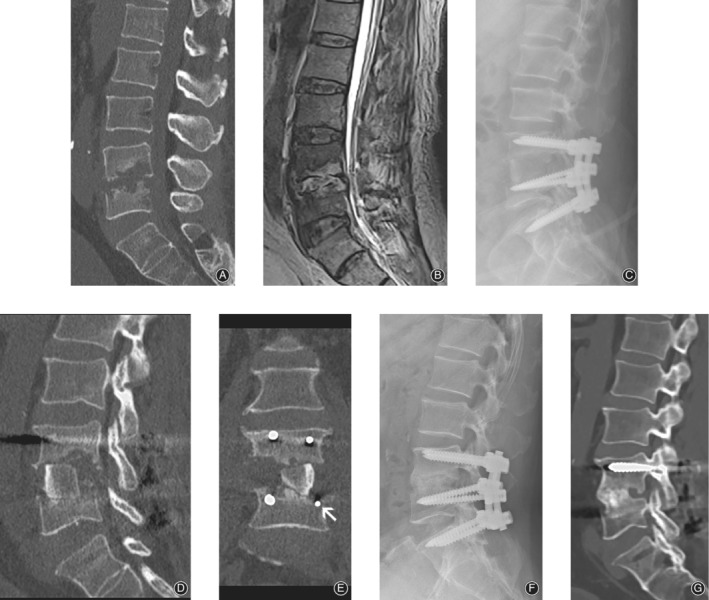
Preoperative CT (A), MRI (B) of a 63‐year‐old man suffering from L_4/_
